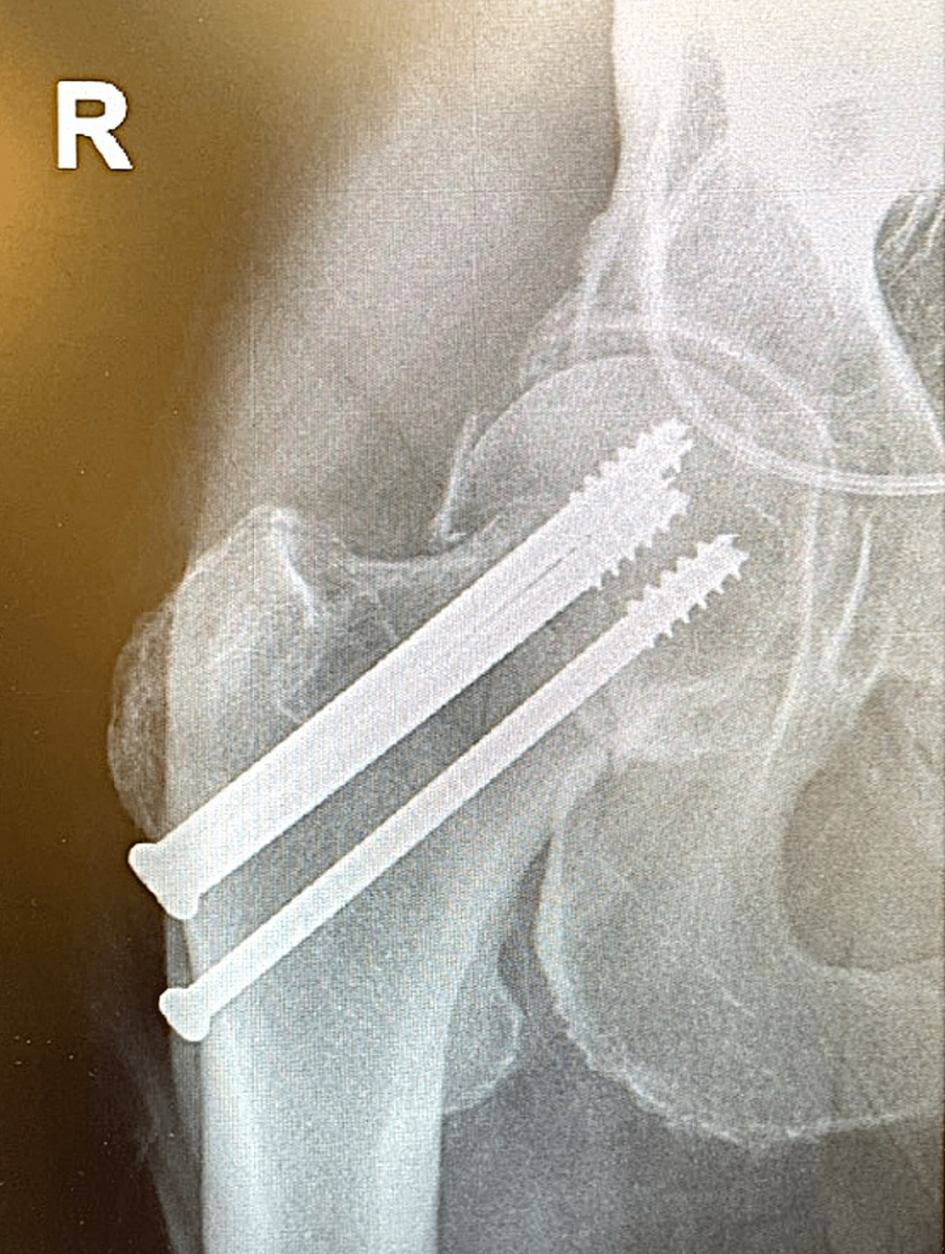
Three weeks post-operative AP radiograph Anterior-posterior (AP) radiograph of the right hip taken three weeks post-operatively shows no displacement or loss of fixation.
